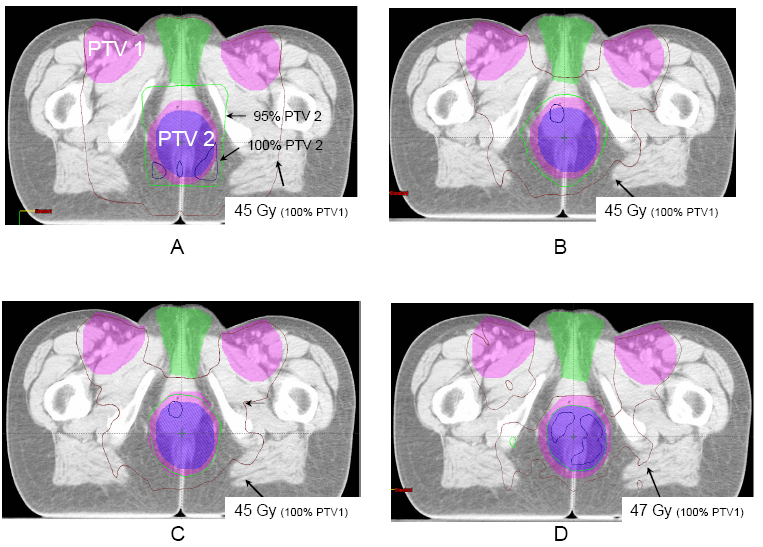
Dose distributions by treatment arm on the same axial CT slice through both target volumes (PTV1 and PTV2) and the external genitalia in a female patient. This CT slice shows the sparing of the genitalia by the 45 Gy isodose curve in arms B, C, and D as compared with arm A.
